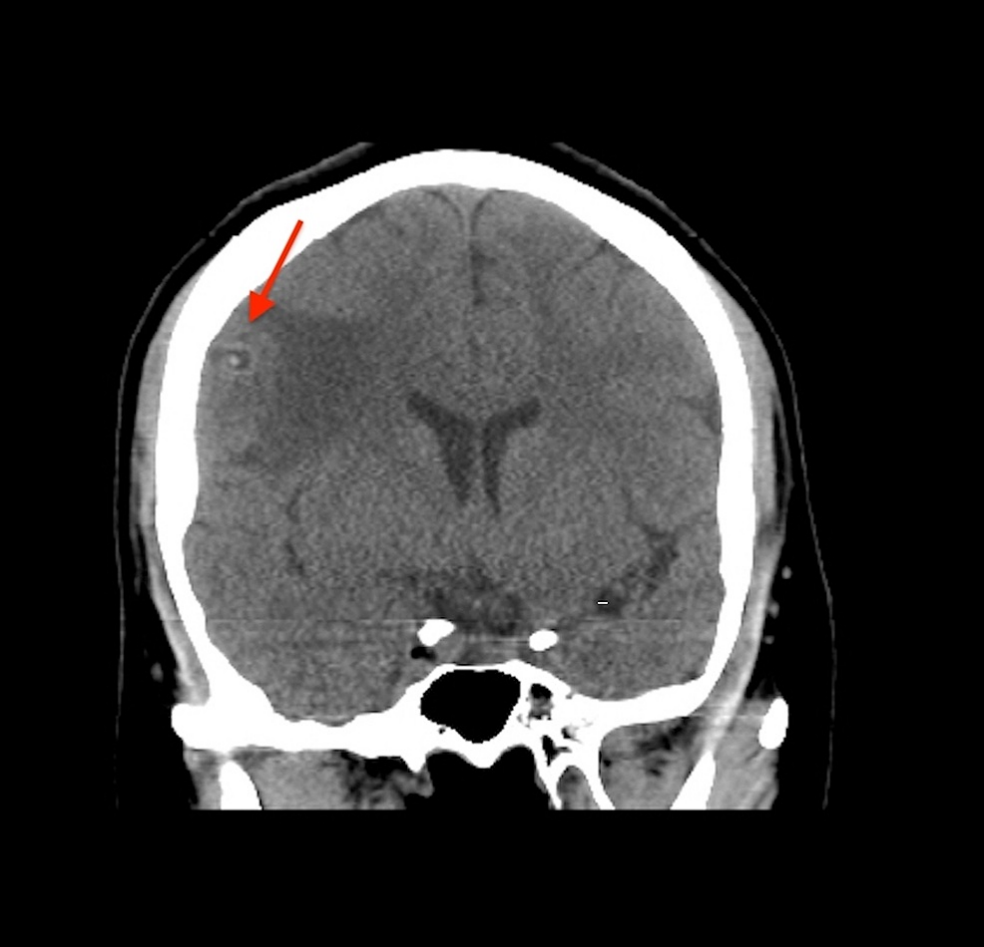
Coronal view CT brain showing ring-like hyperdensity (red arrow) with surrounding vasogenic edema.
